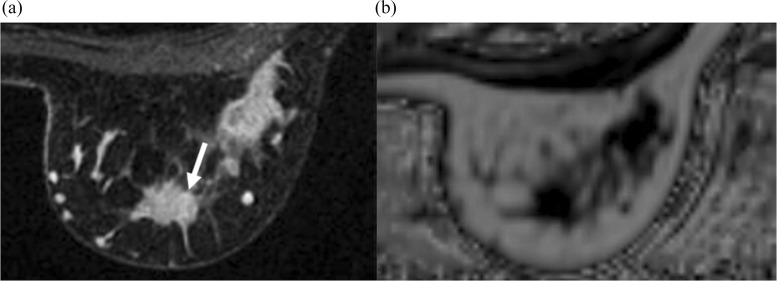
Representative case of breast carcinoma with axillary lymph node metastasis and the high percentage of MIB-1 index. Fat-suppressed post-contrast T1-weighted image (a) shows the enhancing mass (arrow) in the left breast, and the corresponding average peritumoral FFt (76.7) on the fat fraction map (b) and the pTFR (0.84) are low. Reprinted from reference Hisanaga et al.^83^ FFt, fat fraction values; pFTR, peritumoral fat ratio.
